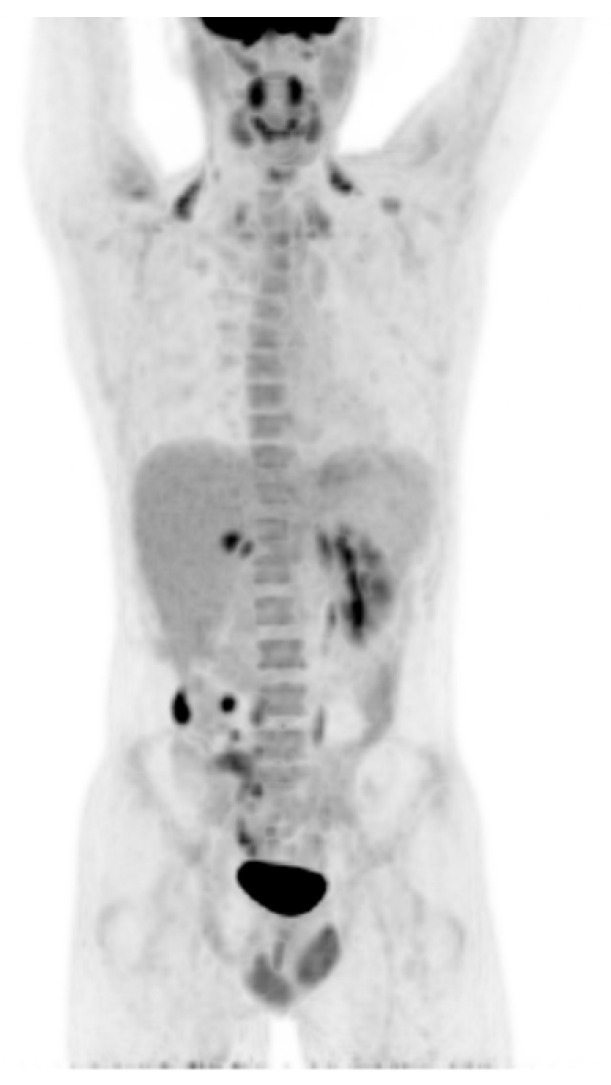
PET-CT confirming first progression, with locoregional lesions, hepatic and retroperitoneal metastasis.
